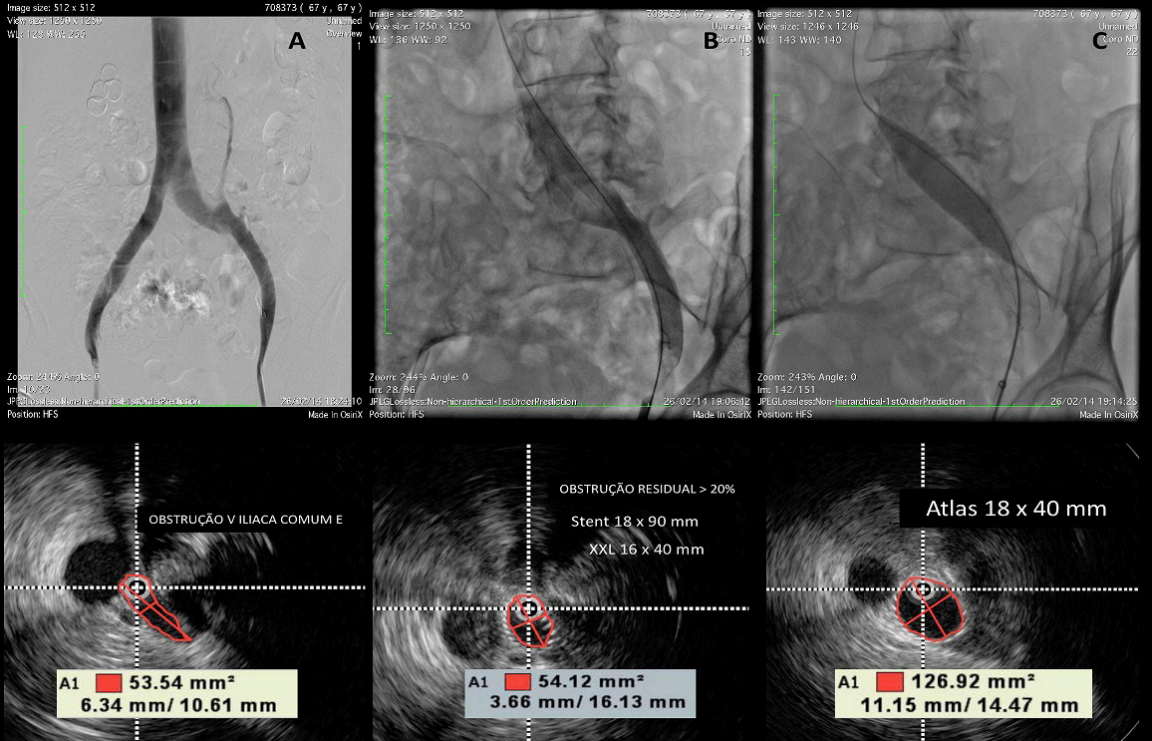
Final control intravascular ultrasound (IVUS). In (A), initial phlebography demonstrating direct and indirect signs of obstruction of the left iliac vein, confirmed by IVUS (area at the point of greatest compression is 53.5 mm^2^). In (B), control phlebography apparently demonstrating a satisfactory result, but not confirmed by IVUS (area at the point of greatest compression is 54.1 mm^2^). In (C), final result after angioplasty with low complacency, high pressure balloon.
